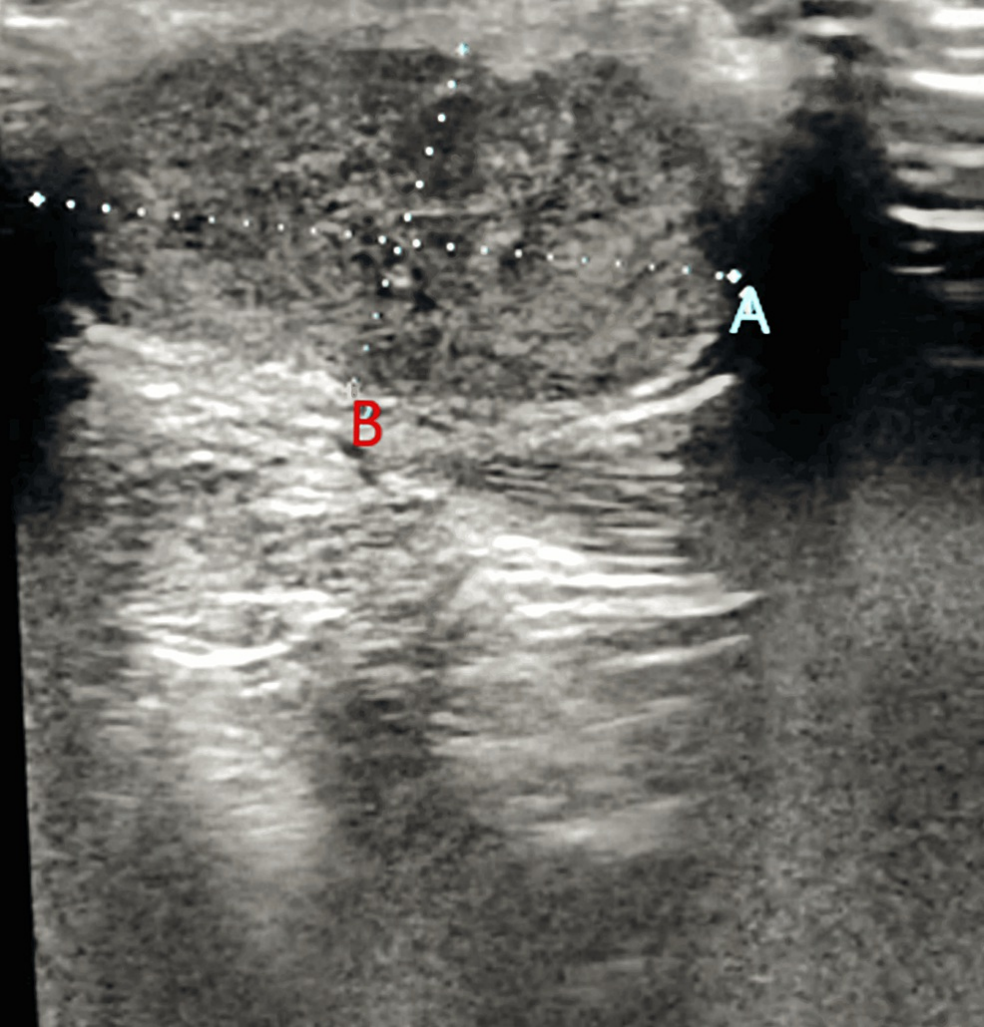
An ultrasound image of the tumor Panes A and B show the length and width of the lesion, respectively.
